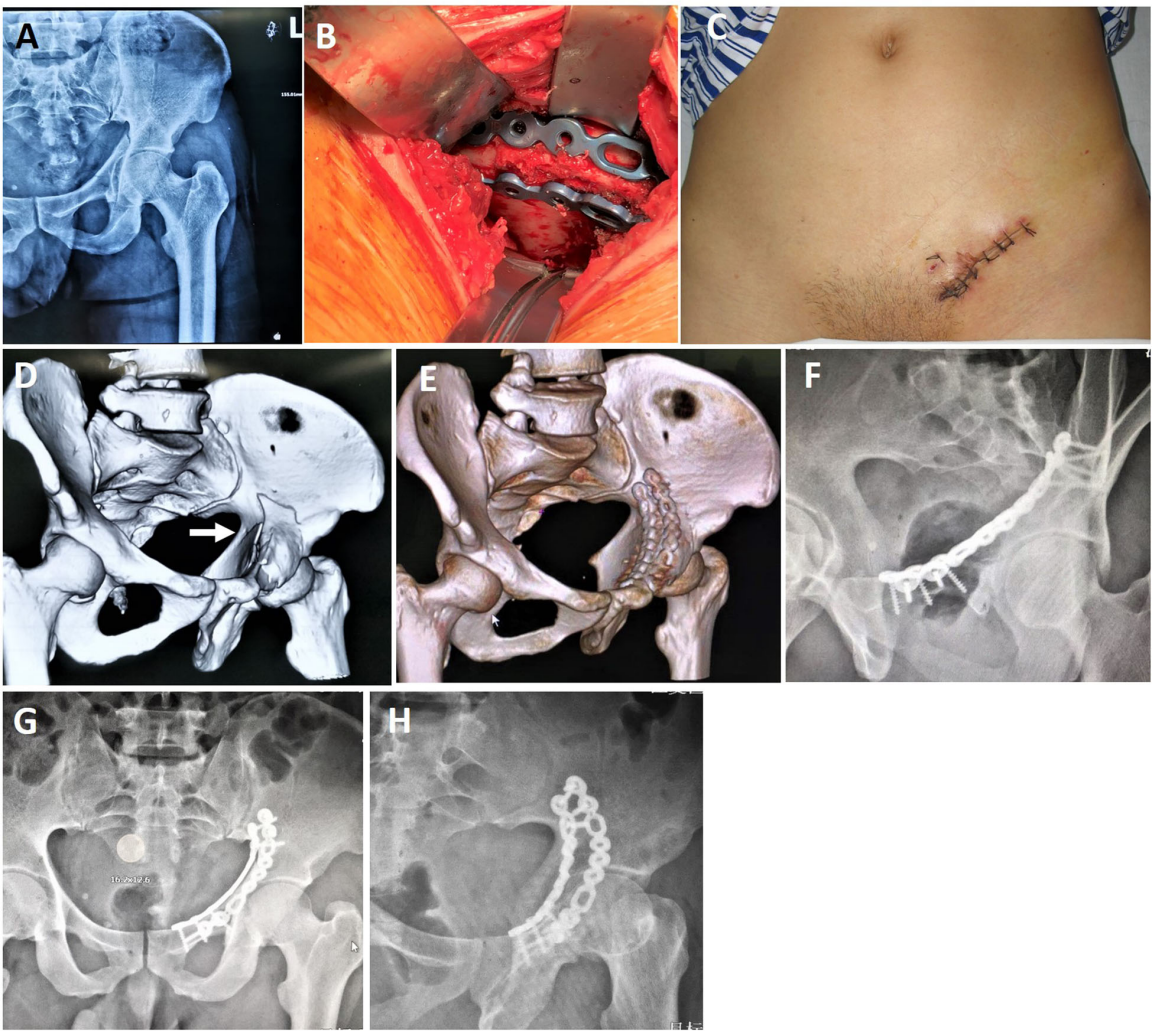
Case 6, a 45-year-old patient who sustained a fracture of the anterior column with medial subluxation. a Preoperative anteroposterior radiograph, b three hooks were used to exposed the anterior wall, pelvic brim and quadrilateral plate, c photograph showing the suture wound after operation, d preoperative 3D CT reconstructions, e postoperative 3D CT reconstruction showing the subluxation of the quadrilateral plate (white arrow), f-h postoperative anteroposterior and Judet oblique views
